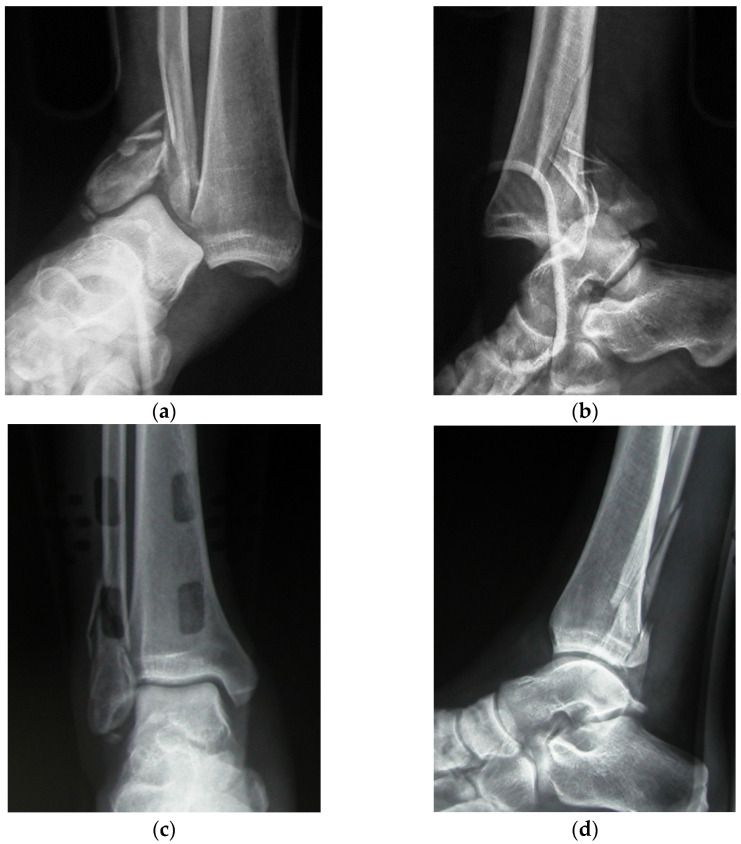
Radiographic examination of a fracture-dislocation of the right ankle in a 32 year-old man (a,b), reduced in the emergency room and temporarily stabilized by a bivalved cast (c,d).
